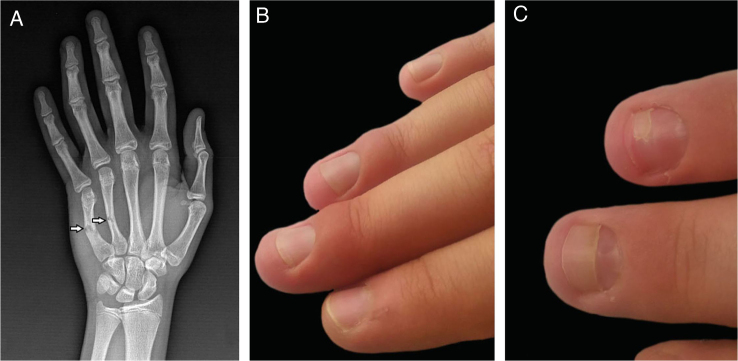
Clinical and radiological images of the second patient. (A), Fourth and fifth metacarpal bone fractures (X-Ray image). (B), Nail fold edema of the third and fourth fingers. (C), Onychomadesis in the two fingers.
